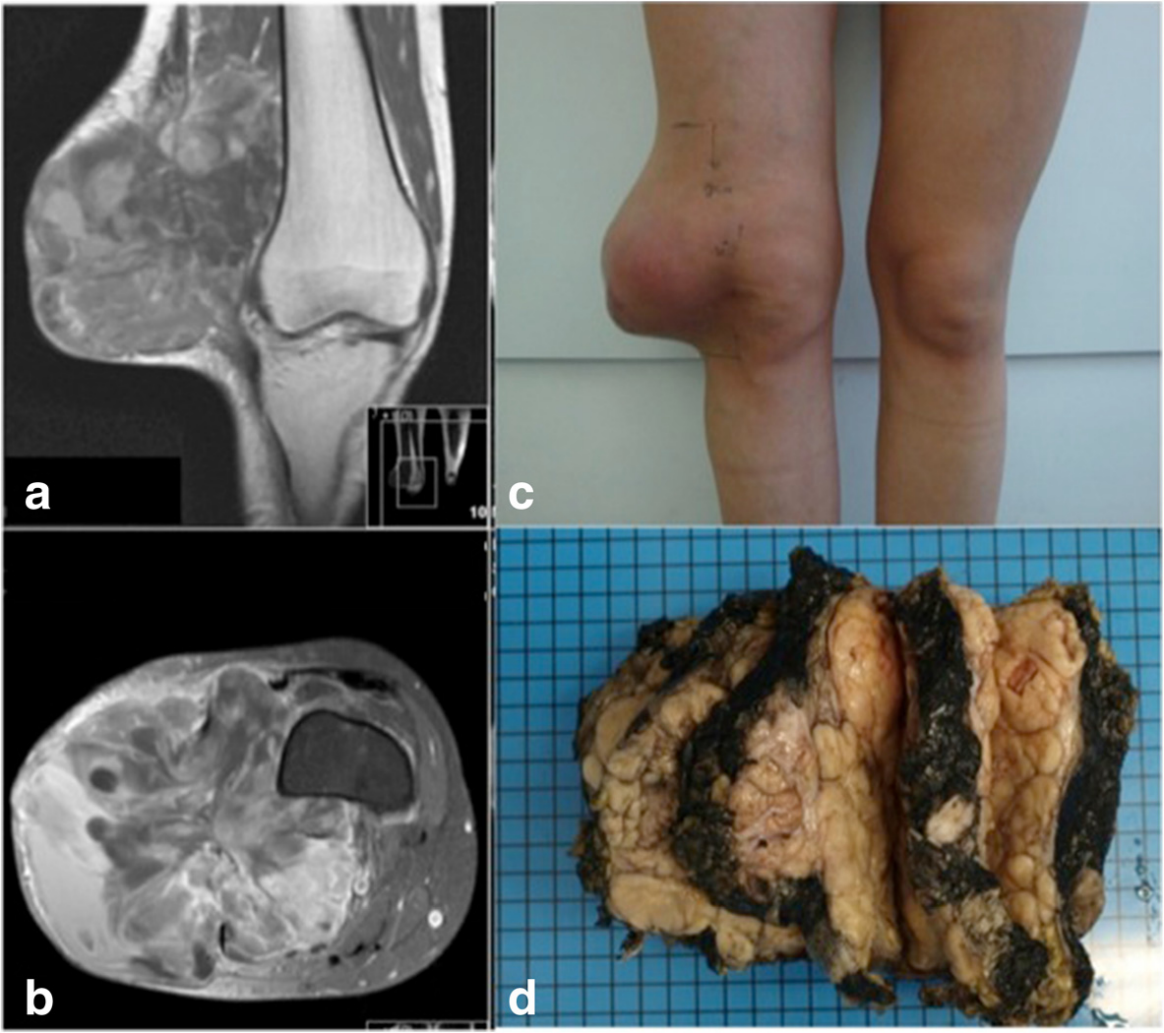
Clinical and radiological appearance of synovial sarcoma: Female patient (30 y. old) with synovial sarcoma of the right knee: a) coronar T1-weighted MRI, b) axial T1-weighted and fat saturated MRI with contrast agent, c) clinical picture bevor limb - salvage surgery, d) cross-sections through the resected tumour.
